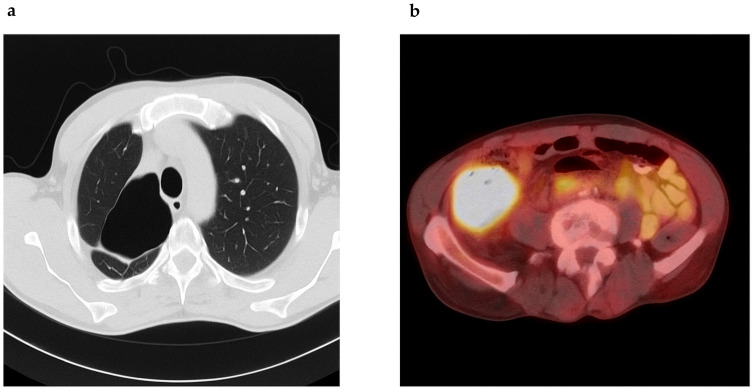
(a) Chest CT reveals a thick-walled cavitary lesion in the apex of the right lung measuring approximately 8.2 × 7.4 cm with other blebs noted in the right upper lobe. (b) PET scan reveals a 7.4 cm transaxial markedly hypermetabolic mass in the ascending colon with SUV max 26.0.
